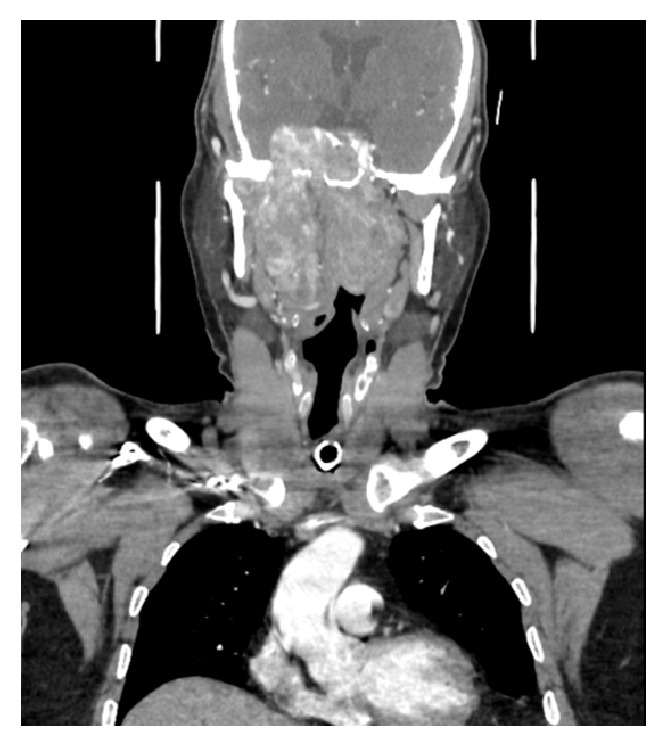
Coronal CT section (after contrast), showing an enhancing mass occupying the nasal cavity, sphenoid sinus, and infratemporal fossa and extending into the parapharyngeal space.
